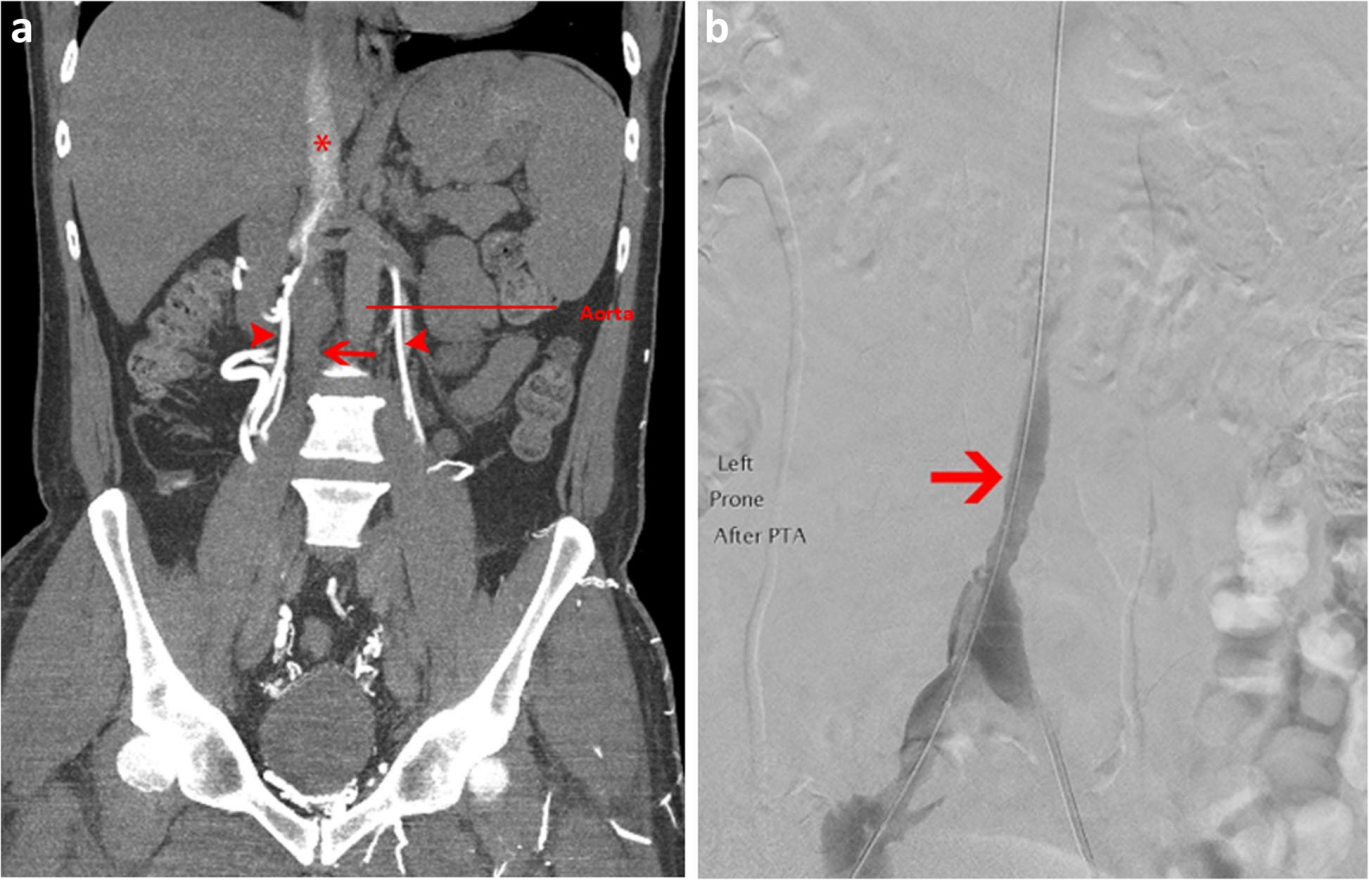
a Coronal CT venogram shows hypoplastic and acutely thrombosed infrarenal IVC (arrow) with contrast taking path of mature iliolumbar and lumbar collateral veins (arrow head). The suprarenal IVC is patent (asterisk). b Venogram after thrombolysis shows restoration of flow to the infrarenal IVC which remains hypoplastic (arrow)
